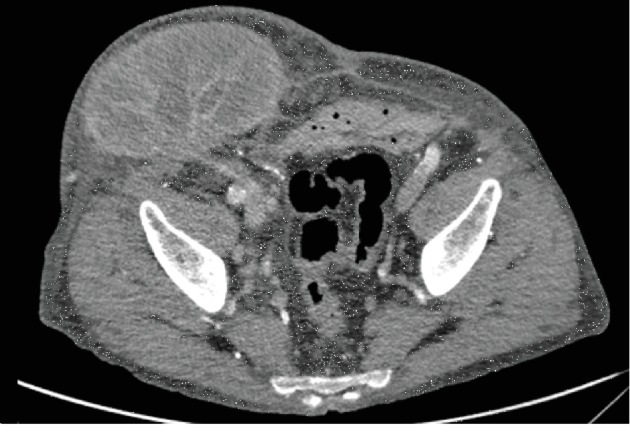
CT scan demonstrating the incisional hernia containing the transplant kidney on the right side of the abdominal wall.
